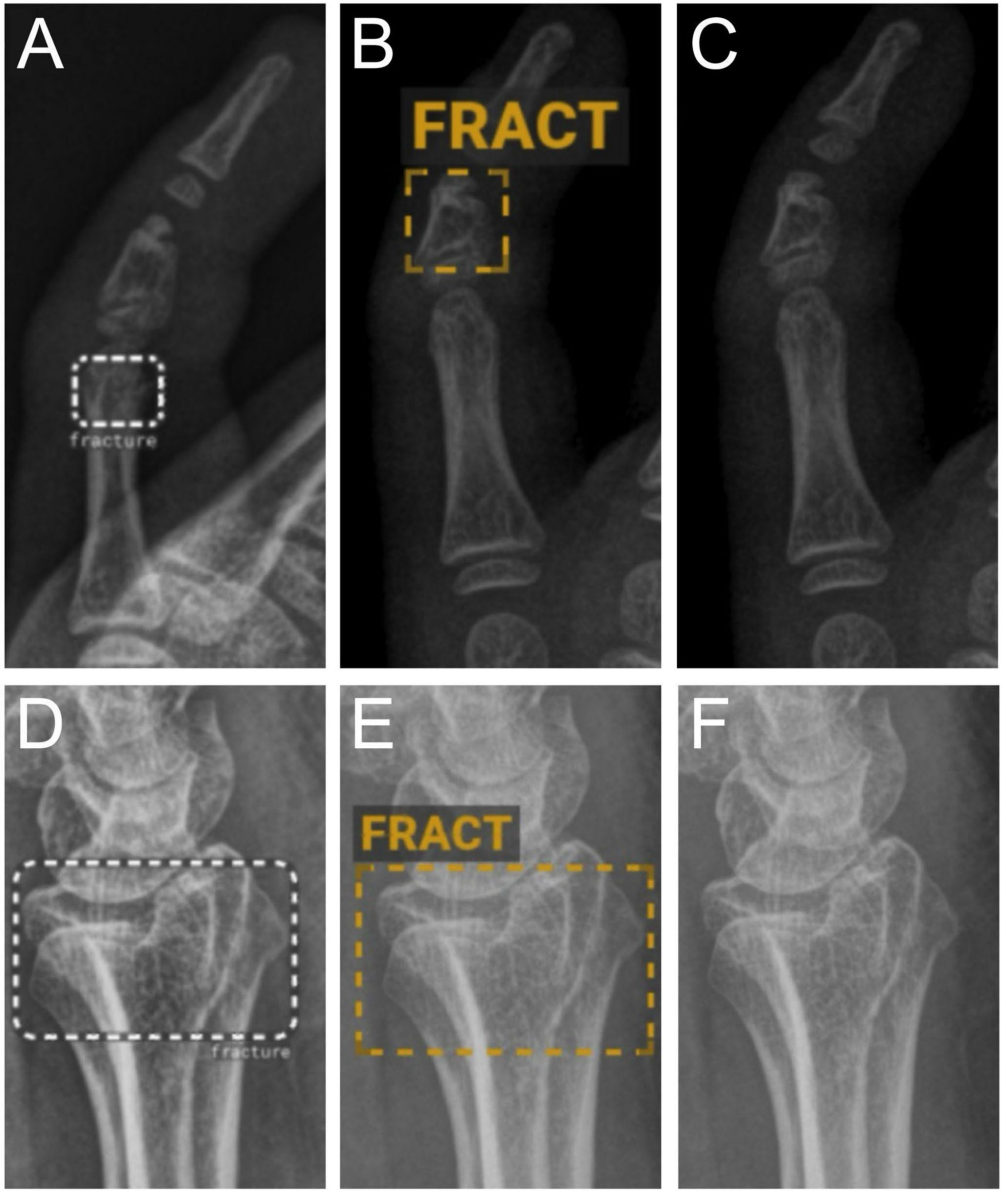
Examples of false positive (FP) findings from both AI algorithms, RBfracture (A, D) and BoneView (B, E), while images C and F show non-annotated radiographs. Of note, A and B represent FP findings in different projections between the AI algorithms. Images A-C correspond to a pediatric patient with no traumatic findings, while images D-F correspond to an adult patient with no traumatic findings in the distal radius
